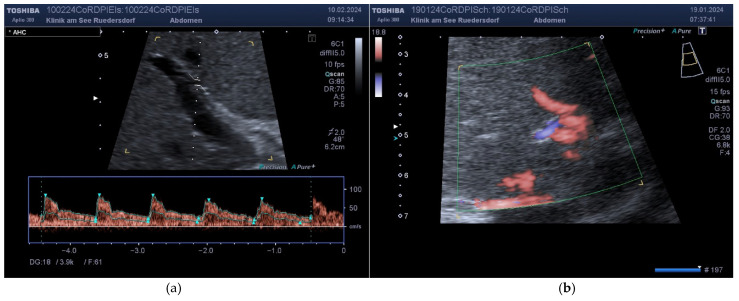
Example of an AHP with a straight course. (a) Doppler with the use of section enlargement. (b) Identification of bifurcation.
